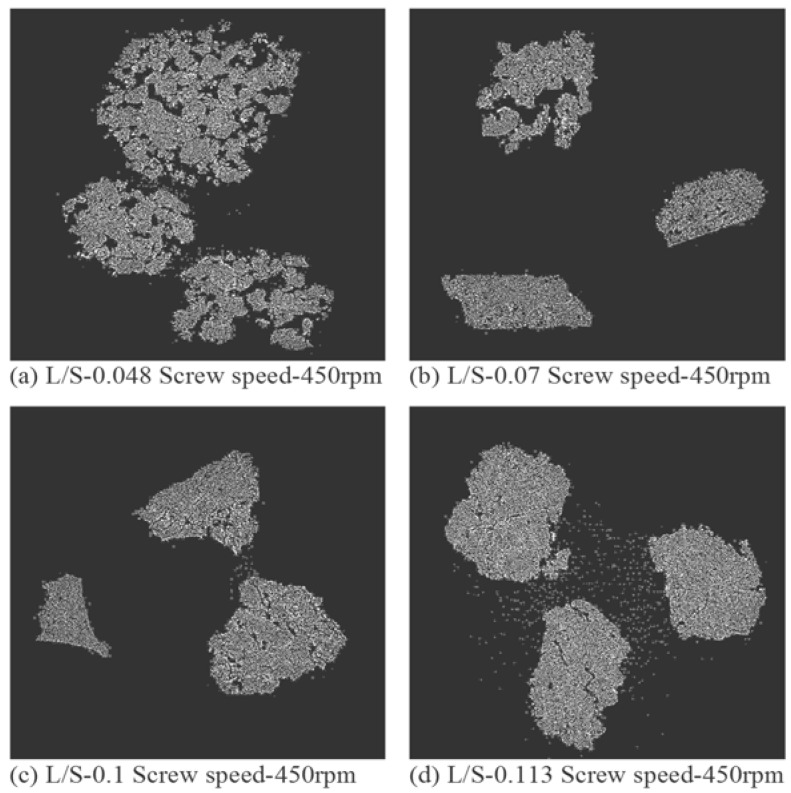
X-ray tomographic images of granules produced using Pearlitol 300DC at varying L/S (screw speed, 450 rpm).
